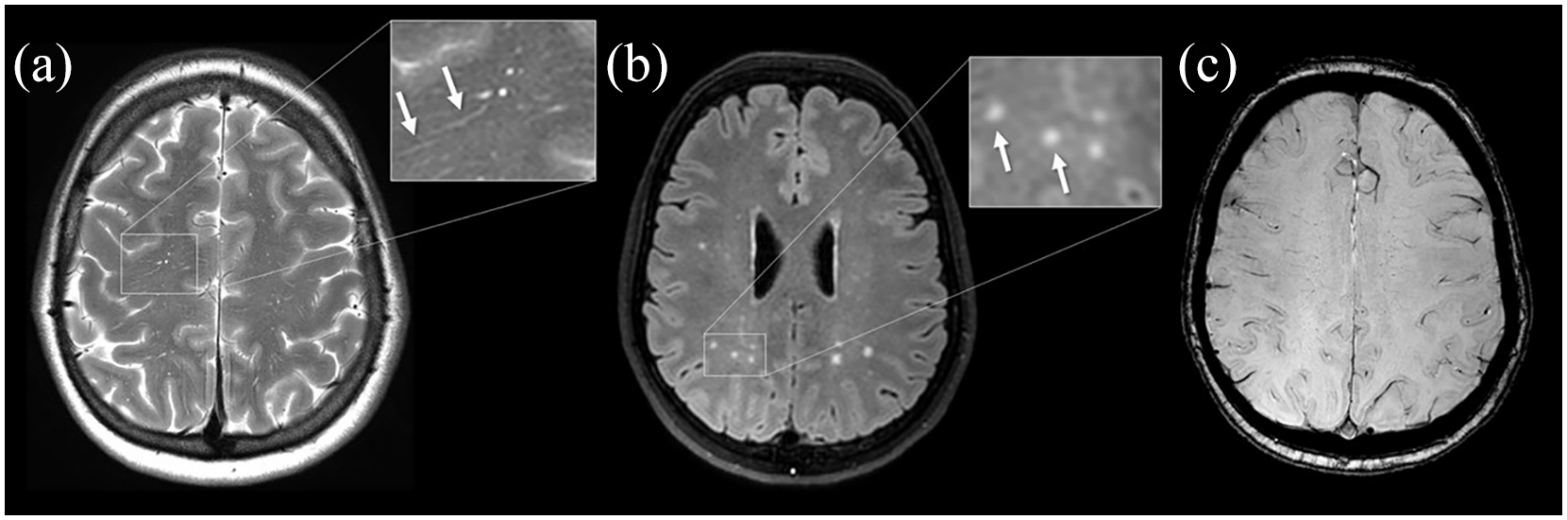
Patient with possible CAA according to the Boston criteria v2.0. Presymptomatic woman with D-CAA with severe (>20 in one hemisphere) visible perivascular spaces in the centrum semiovale on T2-weighted imaging (a, arrows), WMHs in a multispot pattern on FLAIR (b, arrows), and no hemorrhagic MRI markers on SWI (c). This patient fulfills the Boston criteria v2.0 but not the modified Boston criteria v1.5 for possible CAA.
